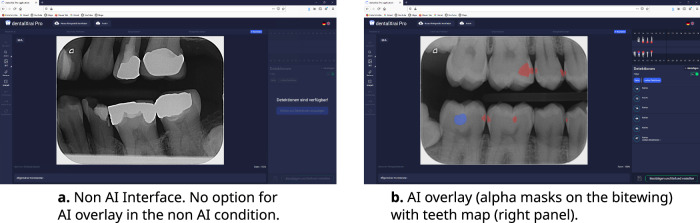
Example of web browser interface with and without AI overlays. Example of the web browser interface with presentation of the bitewing and left panel for image manipulations and right panel presented the AI information for the AI condition (b) or was blank for the non-AI condition (a).
